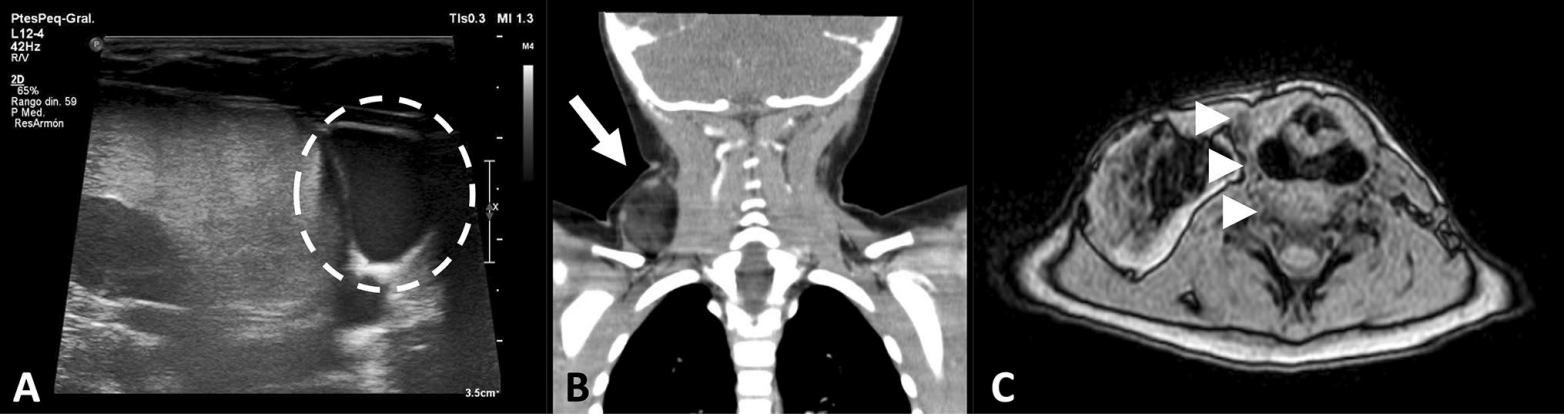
Imaging modalities available for lipomatous tumors. A Hypoechoic, well-circumscribed soft tissue lesion on ultrasound (dotted line). B Hypodense, encapsulated mass on coronal CT with heterogeneous contrast enhancement (white arrow). C Heterogeneous mass with fat signal intensity on axial MR, which displaces adjacent structures (arrow heads)
